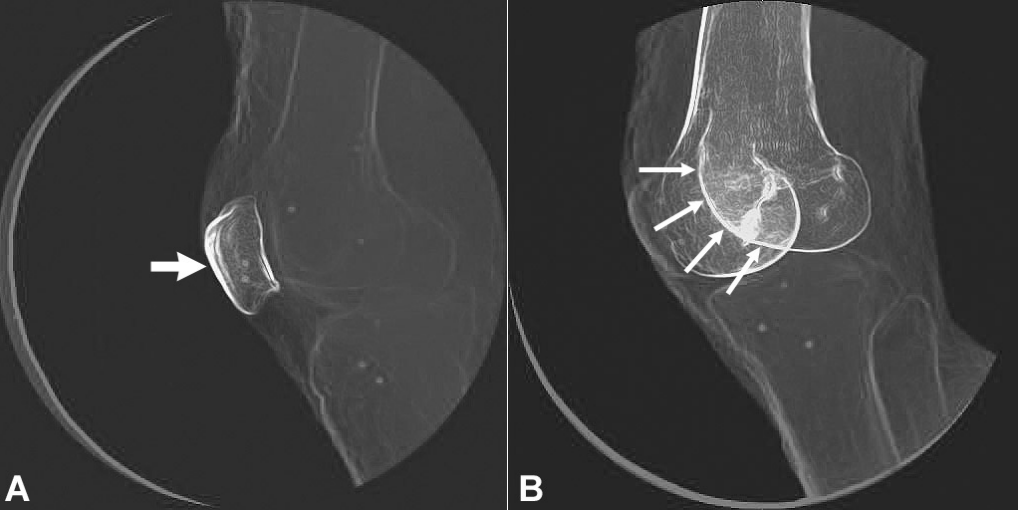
The model-based tracking technique relies upon: A) internal information such as subtle differences in bone density and/or B) the presence of bone edges in an x-ray image that do not necessarily contribute to the outline of a particular bone in all joint positions.
